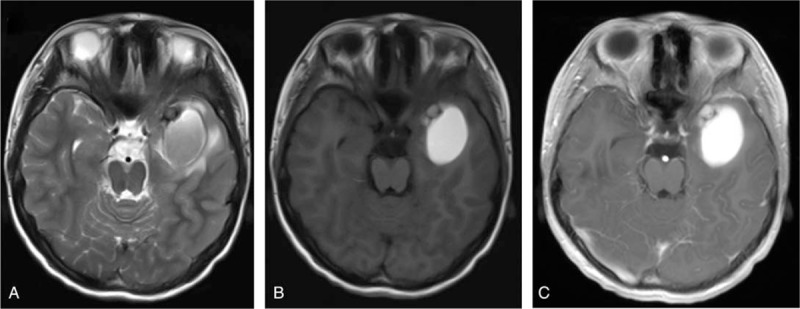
MRI scan of the lesion in the left temporal lobe with (A) iso-signals on T_2_-WI, (B) high signals on T_1_-WI, and (C) significant enhancement after administration of gadolinium.
